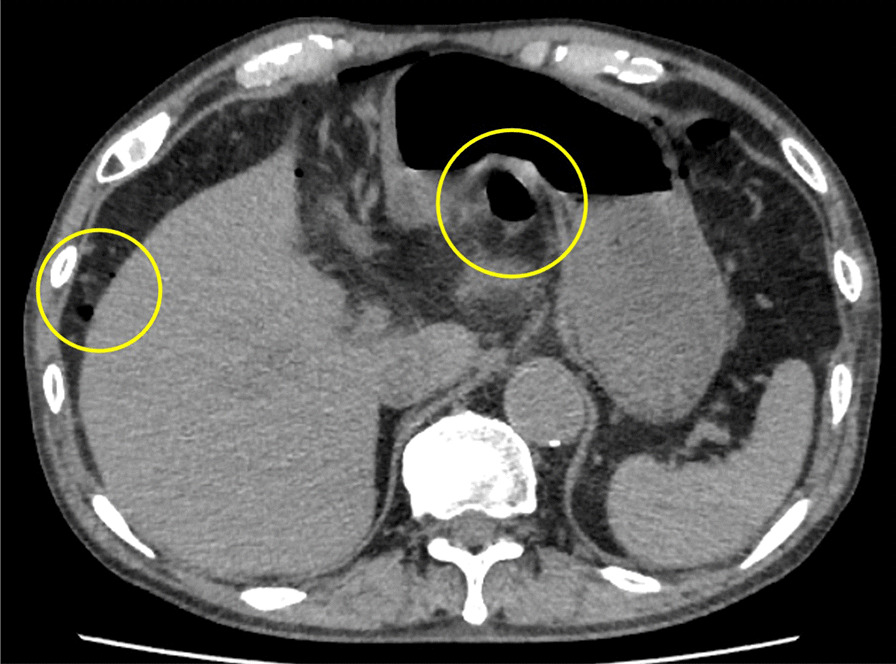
A computed tomography examination revealed the presence of free air in the peritoneal cavity, and a little fluid collection abutting the dorsal area of the stomach. Left circle in the figures shows the free air in the abdominal cavity and right circle shows the fluid collection caused by delayed perforation
